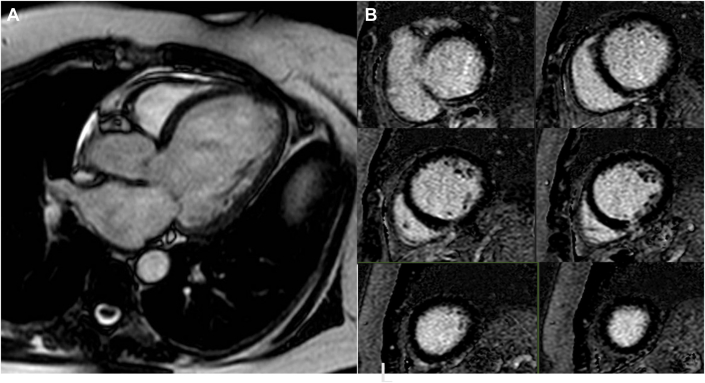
Cardiac Magnetic Resonance Imaging (A) Still frame of cardiac magnetic resonance imaging demonstrating a dilated left ventricle (indexed end diastolic volume of 126 mL/m^2^). (B) Panel of late gadolinium images demonstrates preserved viability with the absence of a scar.
